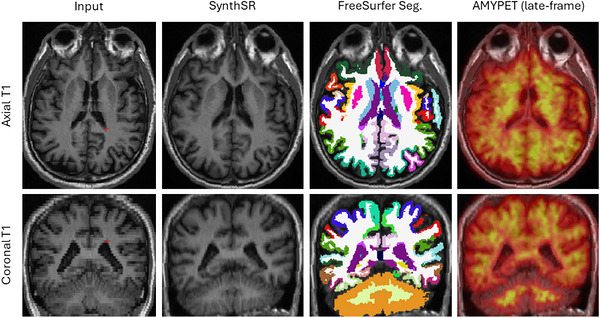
Example of synthetic MRI reconstruction and multimodal co‐registration. Representative example of an externally acquired T1‐weighted MRI (3‐mm of axial slice thickness) on the left, and the corresponding synthetic 1‐mm isotropic MPRAGE‐like reconstruction generated using SynthSR. The Desikan‐Killiany cortical parcellation obtained from FreeSurfer processing and the co‐registered late‐frame amyloid PET image are shown as overlays. The first row displays axial views and the second row coronal views.
